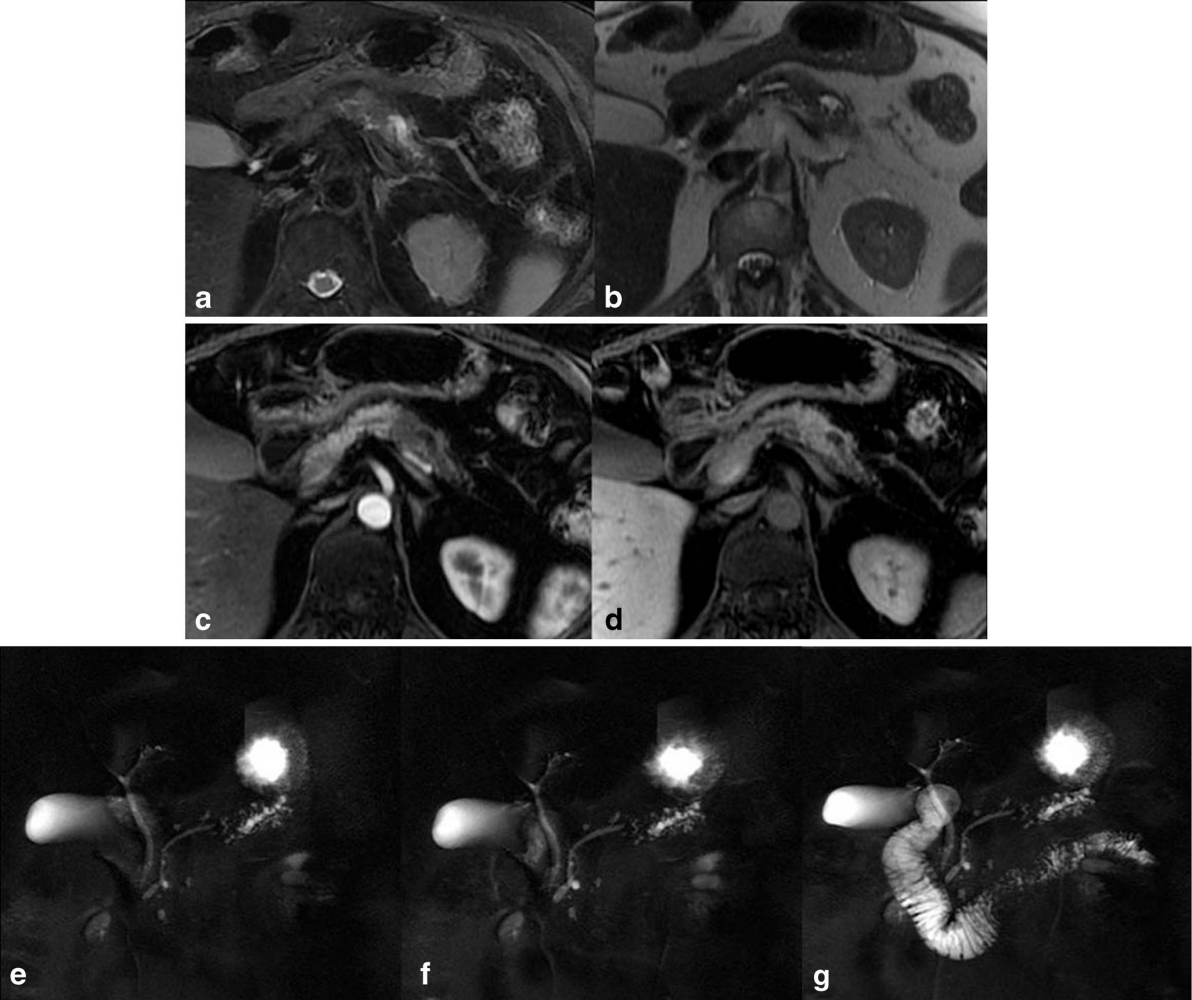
a–g Ductal adenocarcinoma. Axial T2-weighted (a, b) and axial post-contrast T1-weighted images (c, d) show a small lesion at the level of the pancreatic body with an abrupt cut-off of the main pancreatic duct, better visualised on MRCP (e). After secretin stimulation (f, g) there is a persistent stenosis, without the duct-penetrating sign
